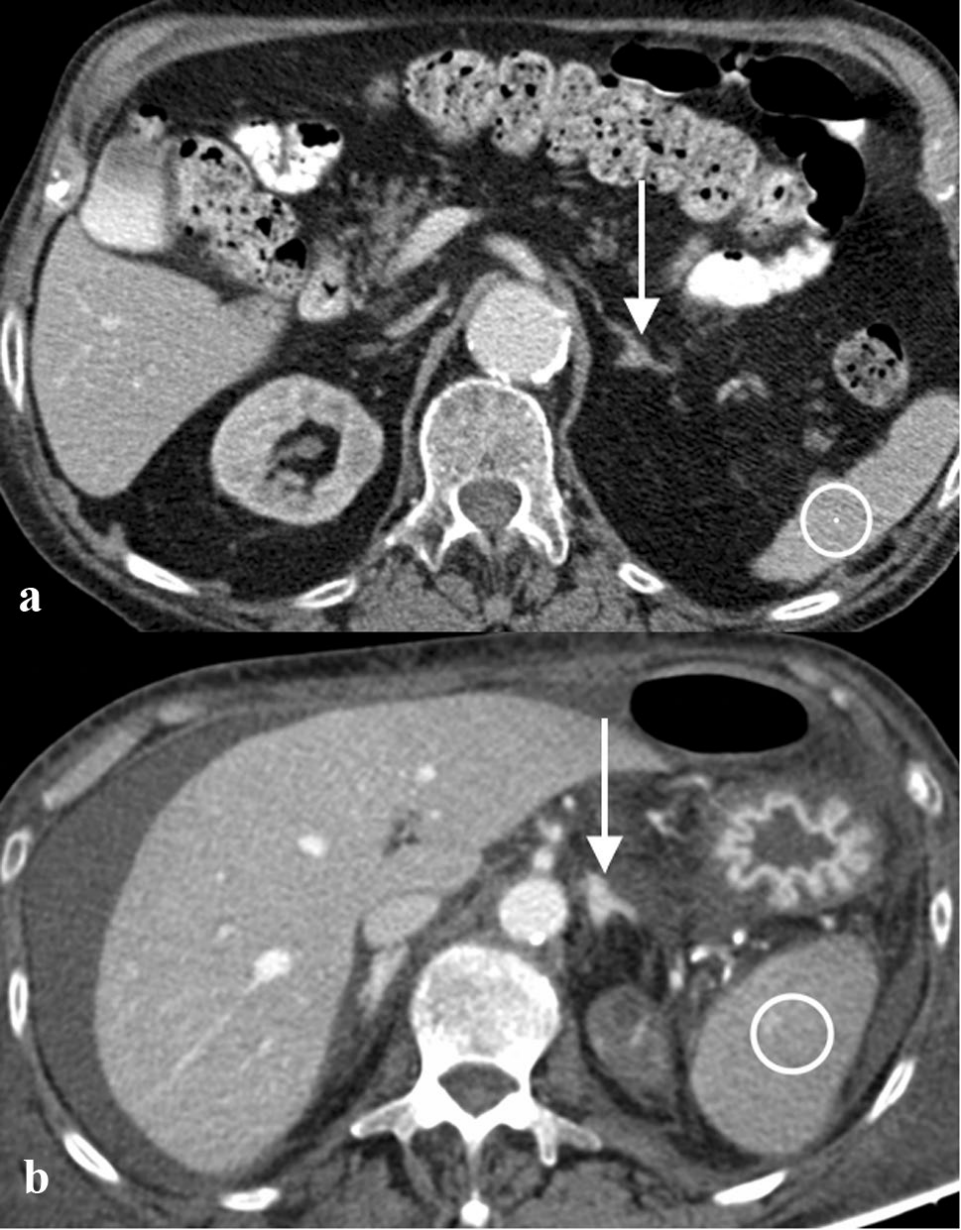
a Abdominal CT scan of an 83-year-old woman with aortic dissection of the ascending aorta. White-colored arrow point to the left hyperdense adrenal gland (average HU adrenal gland: 75); white-colored circle within the spleen (averaged HU spleen: 95; adrenal gland-to-spleen ratio  ≈ 0.79). The patient survived. b Abdominal CT scan of a 78-year-old male patient with septic shock. White-colored arrow point to the left hyperdense adrenal gland (average HU adrenal gland: 160); white-colored circle within the spleen (averaged HU spleen: 93; adrenal gland-to-spleen ratio: ≈ 1.72). The patient died within 24 h after the imaging
